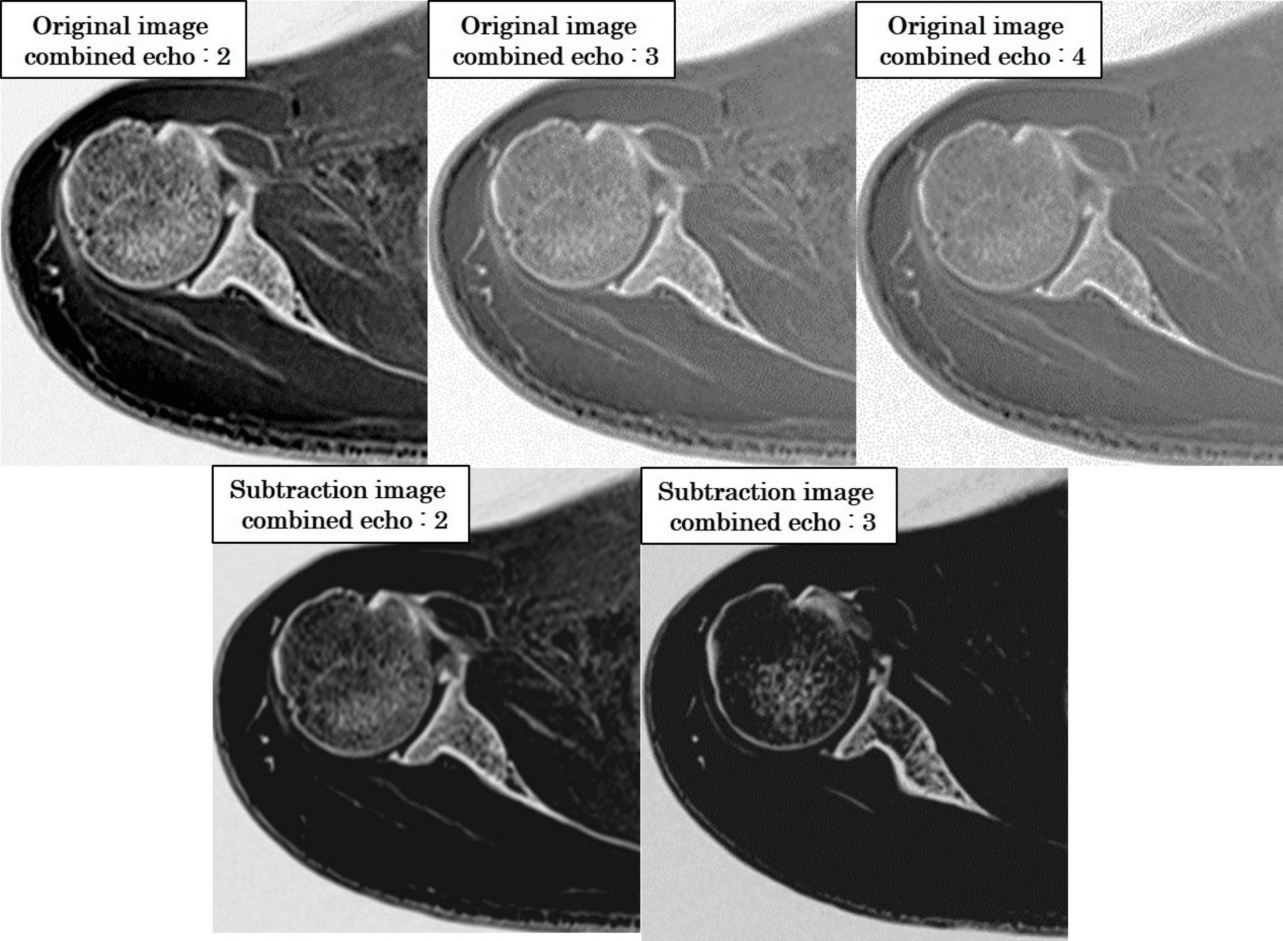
Effect of echo combination and subtraction processing on image contrast in CT-like MRI Grayscale-inverted images generated using two, three, and four combined echoes, as well as subtraction images based on two- and three-echo combinations. The two-echo subtraction condition yielded the highest bone-to-muscle contrast and the most distinct anatomical delineation. In comparison, three- and four-echo images showed progressively lower contrast due to signal averaging, and three-echo subtraction images also exhibited diminished tissue differentiation. Visual assessment confirmed the superiority of the two-echo subtraction approach for CT-like image quality.
